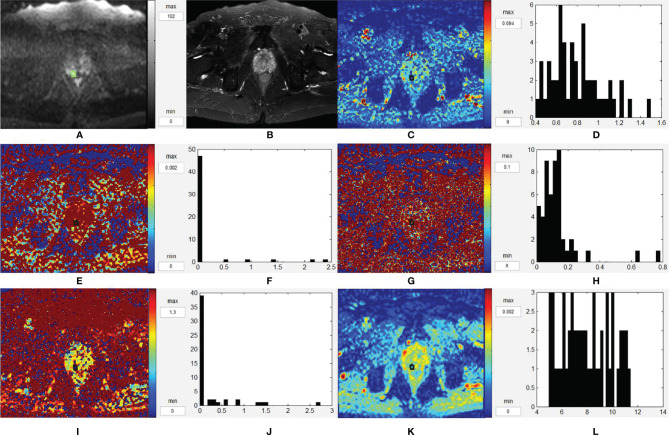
Representative images from a 63-year-old BPH/prostatitis patient, as outlined on the images. (A–L) Axial DWI (*b* = 1,500 s/mm^2^), axial T2WI, *D* map, histogram of *D*, *D map, histogram of *D, *f* map, histogram of *f*, *K* map, histogram of *K*, ADC map, and histogram of ADC, respectively. BPH, benign prostatic hyperplasia; T2WI, T2-weighted images; DWI, diffusion-weighted images; ADC, apparent diffusion coefficient.
